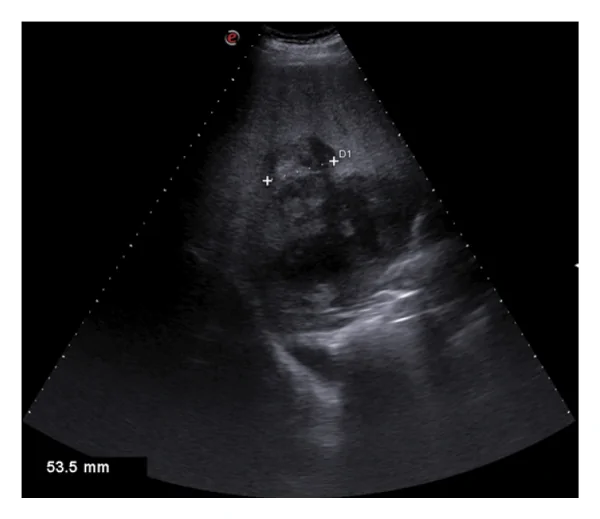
Heterogeneous, mixed‐echogenic hepatic cavities involving segments V and VII on ultrasonography.
